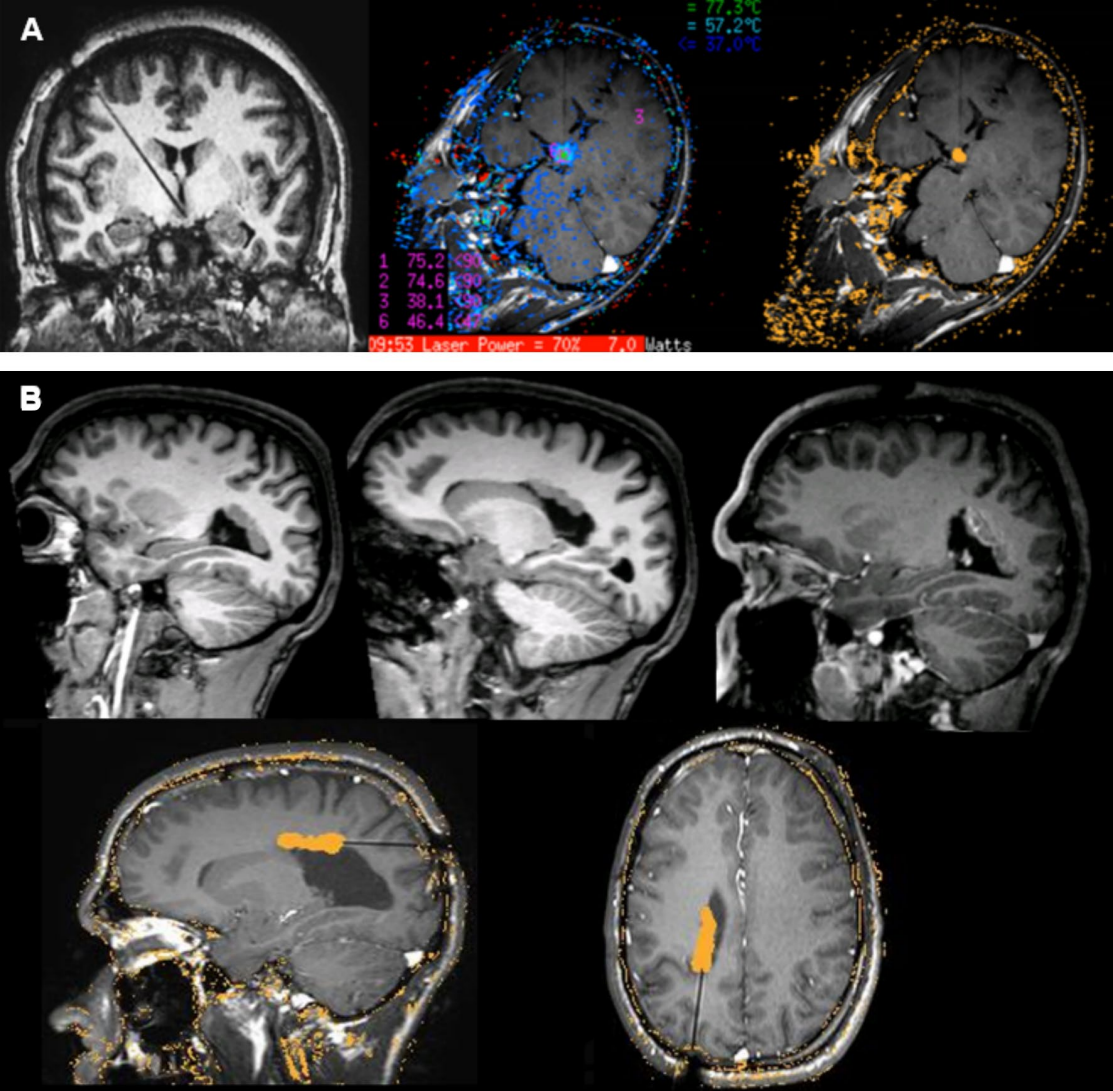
Monitoring images during the ablation procedure. T1 sequence confirming correct fiber placement, temperature monitoring on thermal map and estimated ablation volume in a HH case (A). C-shaped PVH case treated with a two-time approach. Presurgical sagittal T1 MRI, final contrast-MRI sequence post-ablation after first approach, and estimated ablation volume during the second procedure (B)
